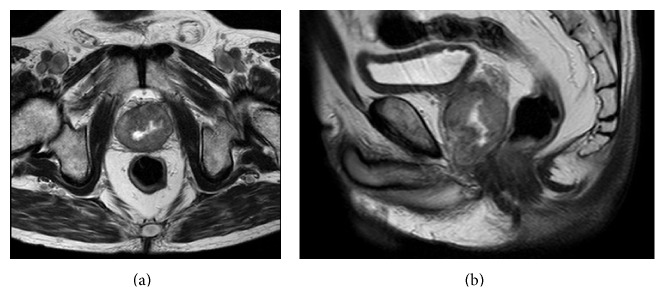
Pelvic MRI ((a) axial and (b) sagittal) showed a lobular lesion, whose vicinity edge is irregular due to fluid collection in the left lobe of his prostate.
